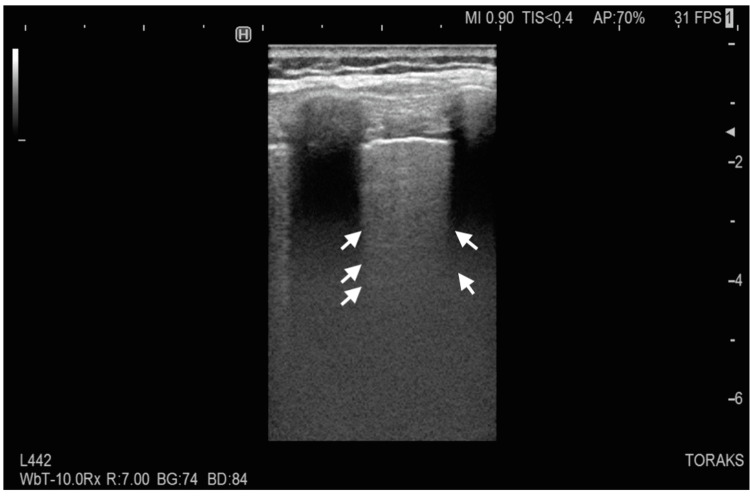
Lung ultrasound of the left lower BLUE point The figure demonstrates no pleural sliding. White arrows indicate areas with coalescent B-lines, called white lung.
